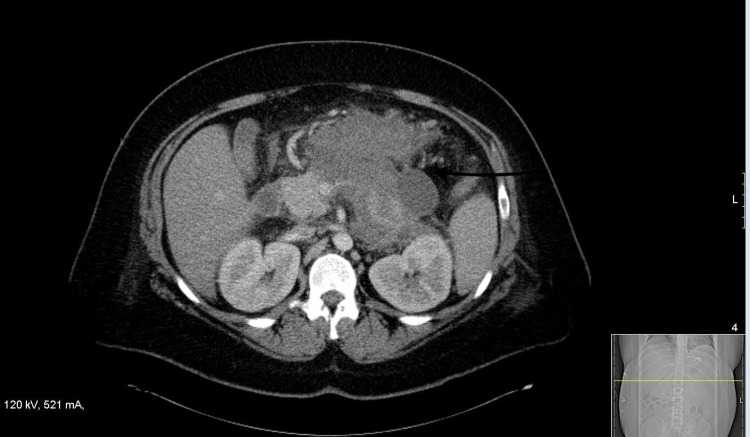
CT scan of the abdomen significant for extensive hyperdense peripancreatic fluid, consistent with hemorrhagic pancreatitis.
